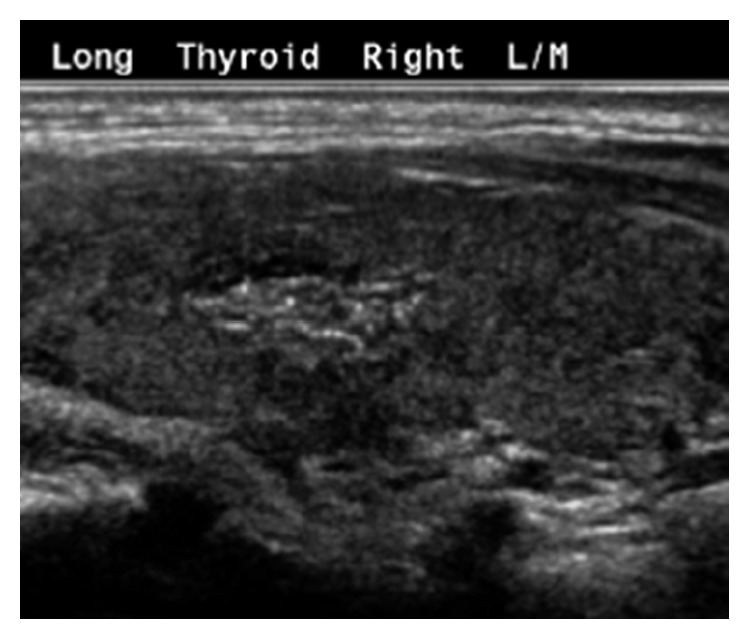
Ultrasound finding showing a 1.3 × 1.7 × 1.1 cm focal area of ill-defined echogenicity in the midright lobe with ill-defined margins, linear echogenic areas, and internal vascularity.
